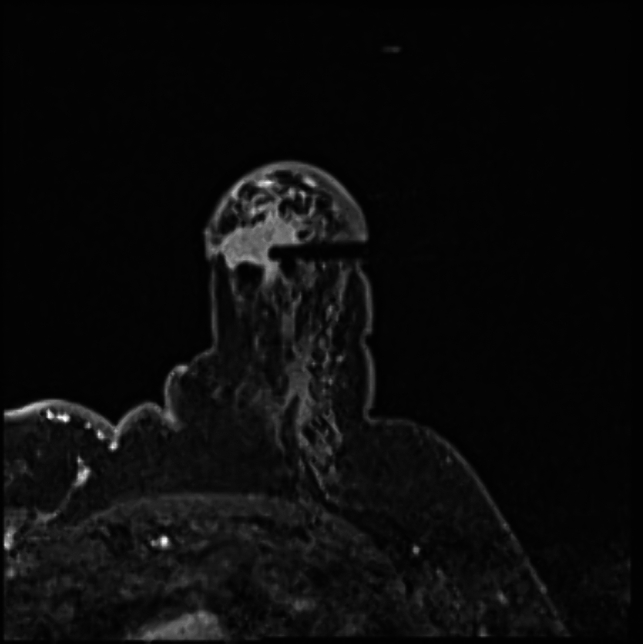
The T1-weighted sequence performed at the end of the sampling procedure reveals the presence of a hematoma at the biopsy site
